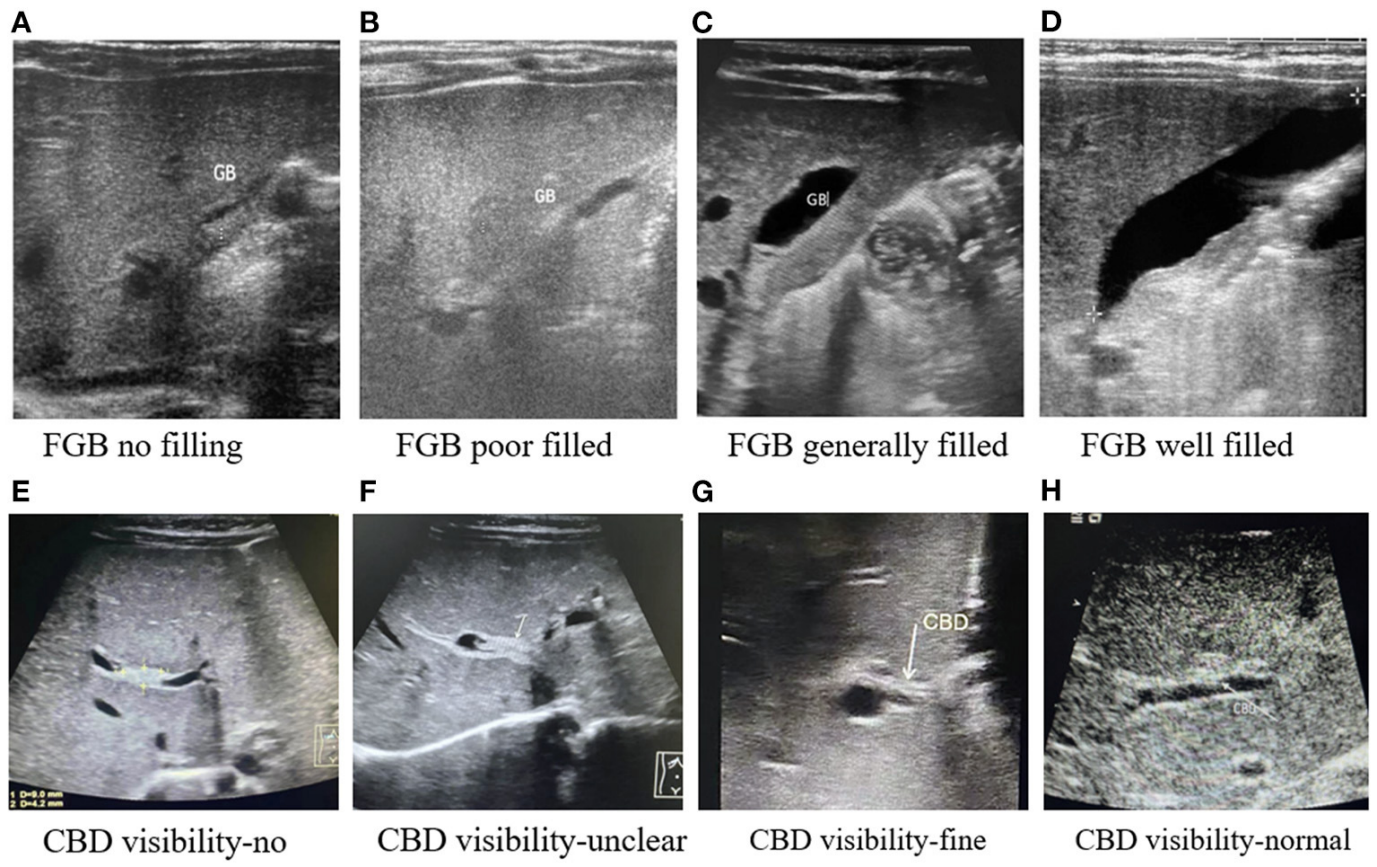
The classification of fasting gallbladder filing status and common bile duct visibility in ultrasonography from our center. (A) FGB no filling. (B) FGB poor filled. (C) FGB generally filled. (D) FGB well-filled. (E) CBD visibility-no. (F) CBD visibility-unclear. (G) CBD visibility-fine. (H) CBD visibility-normal.
